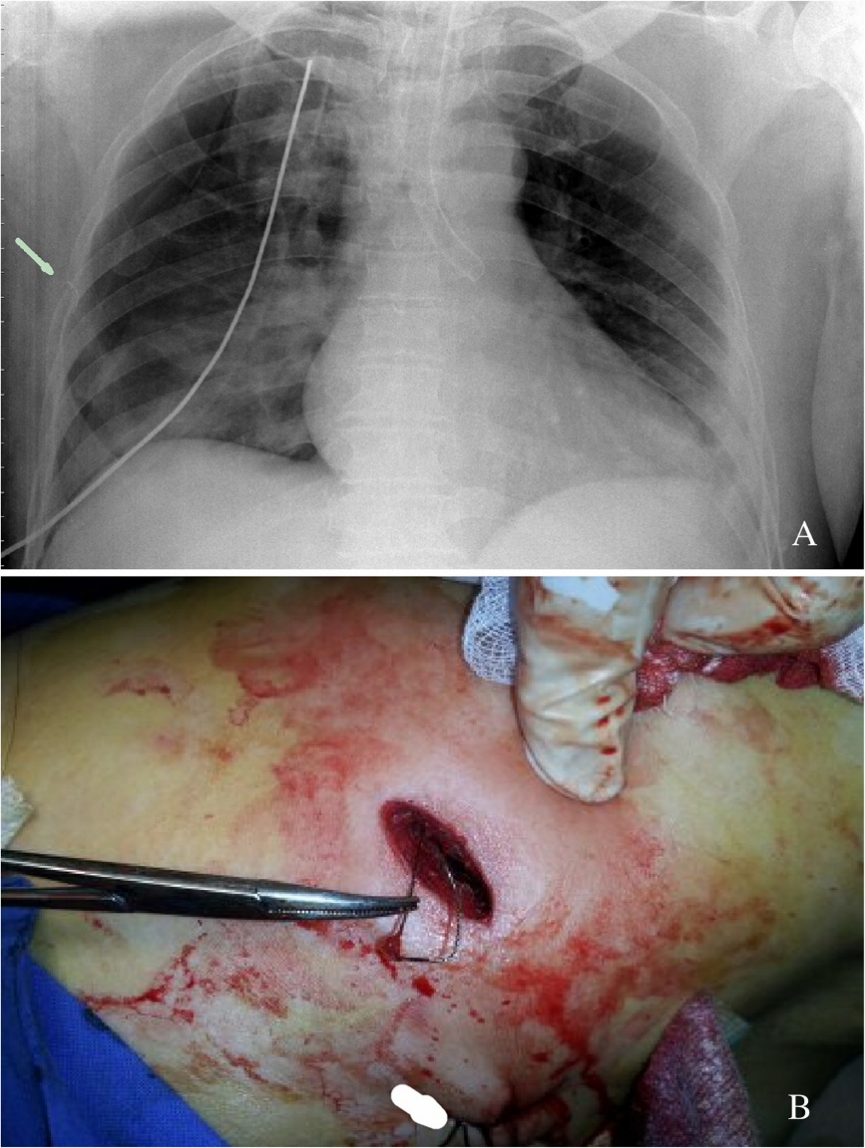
The 'missing' wire was localized and removed. (A) Bed-side X-ray showing the wire (arrow) twisted in the lateral chest wall; (B) The wire was removed through another incision just at the point of puncture posterior to the manipulation hole (arrow).
